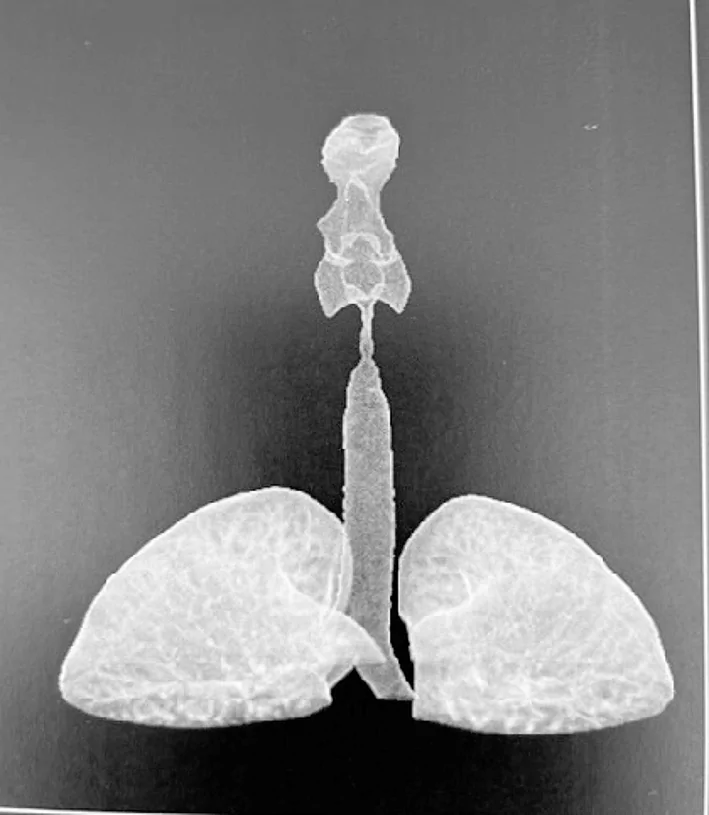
Helicoidal computed tomography showing tracheal stenosis in a patient with RP (Author’s personal archive)
